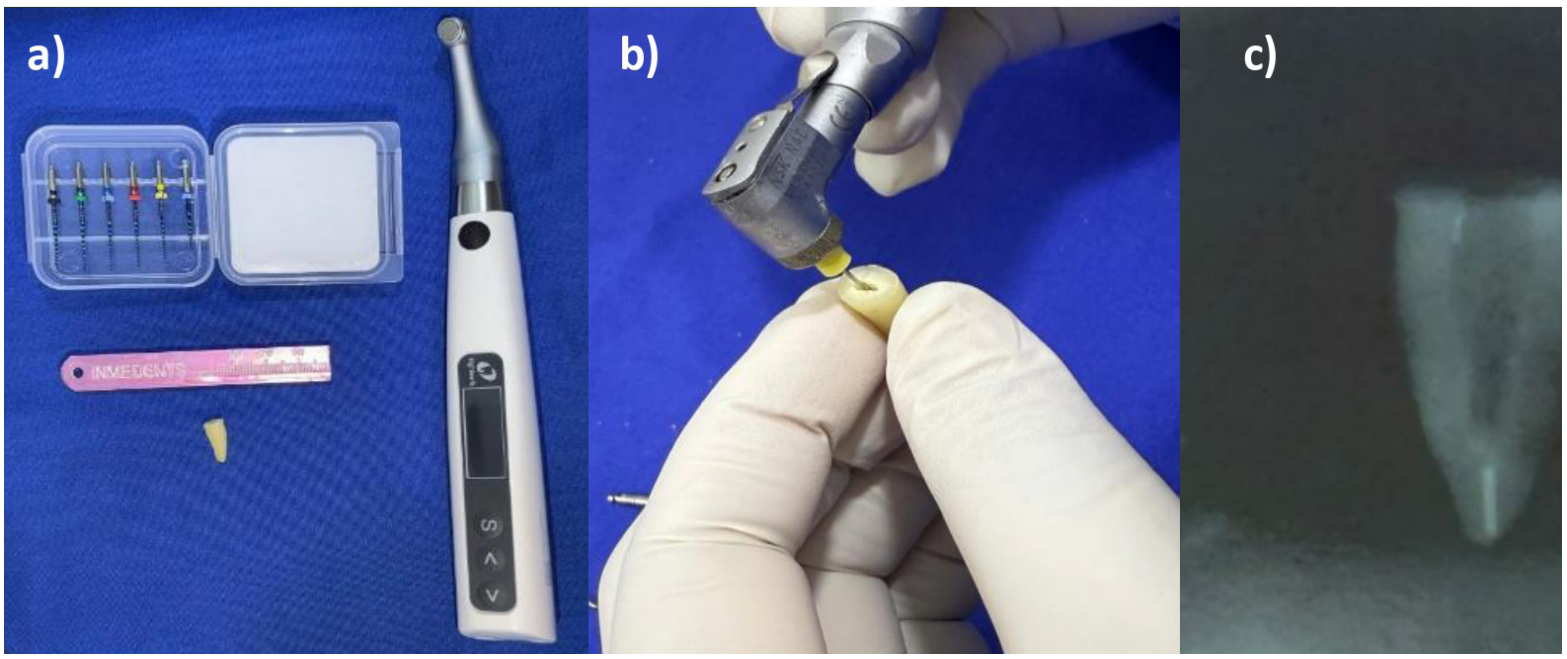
a) Root canal preparation treatment, b) Obturation removal, c) Radiographic evaluation of the spacing for the post.
